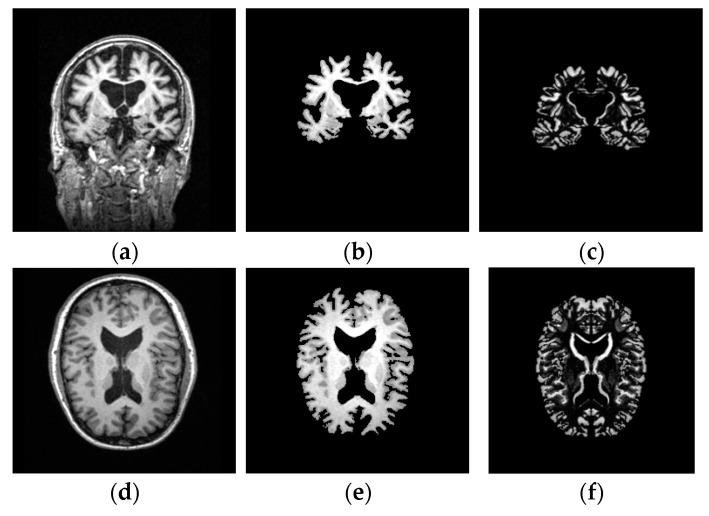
T1-waited brain MRI processing in 2 axes of symmetries: (a) Original image in the coronal plane; (b) Segmented image of the brain tissues in the coronal plane; (c) Image asymmetry in the coronal plane; (d) Original image in the axial plane; (e) Segmented image of the brain tissues in the axial plane; (f) Image asymmetry in the axial plane.
